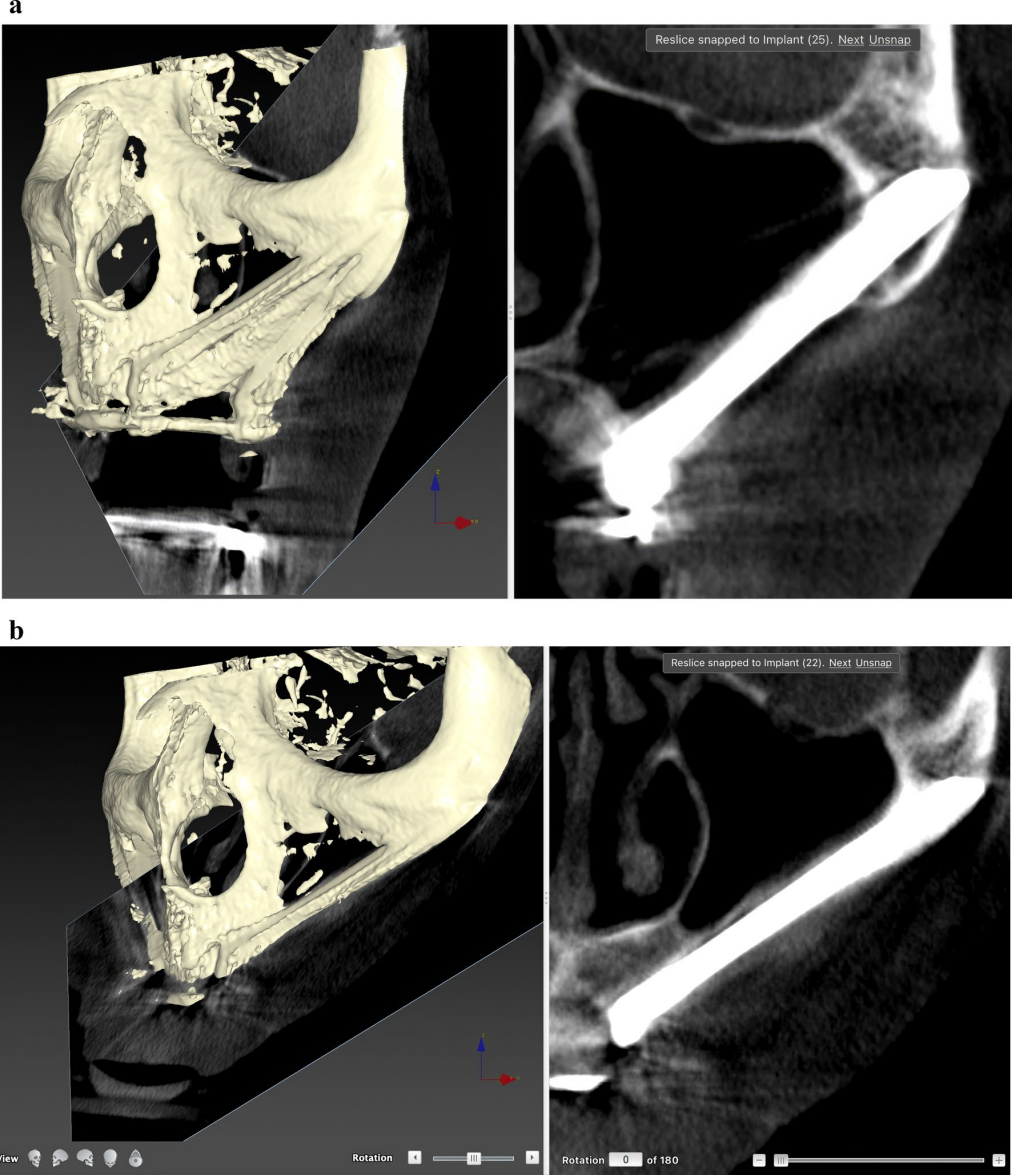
a Oblique CBCT slice taken in the control of the Straumann ZAGA Flat implant placed in the left posterior position in Fig. 1. The image, taken using DTX Studio Implant software, is to be compared with the one in Fig. 2a before the placement of the ZI. This will enable us to understand the condition of the implant and the possible variations in the adjacent structures. b Oblique CBCT slice taken in the control of the Straumann ZAGA Round implant placed in the left anterior position in Fig. 1. The image, taken using DTX Studio Implant software, is to be compared with the one in Fig. 3 before the placement of the ZI. This will enable us to understand the condition of the implant and the possible variations in the adjacent structures
